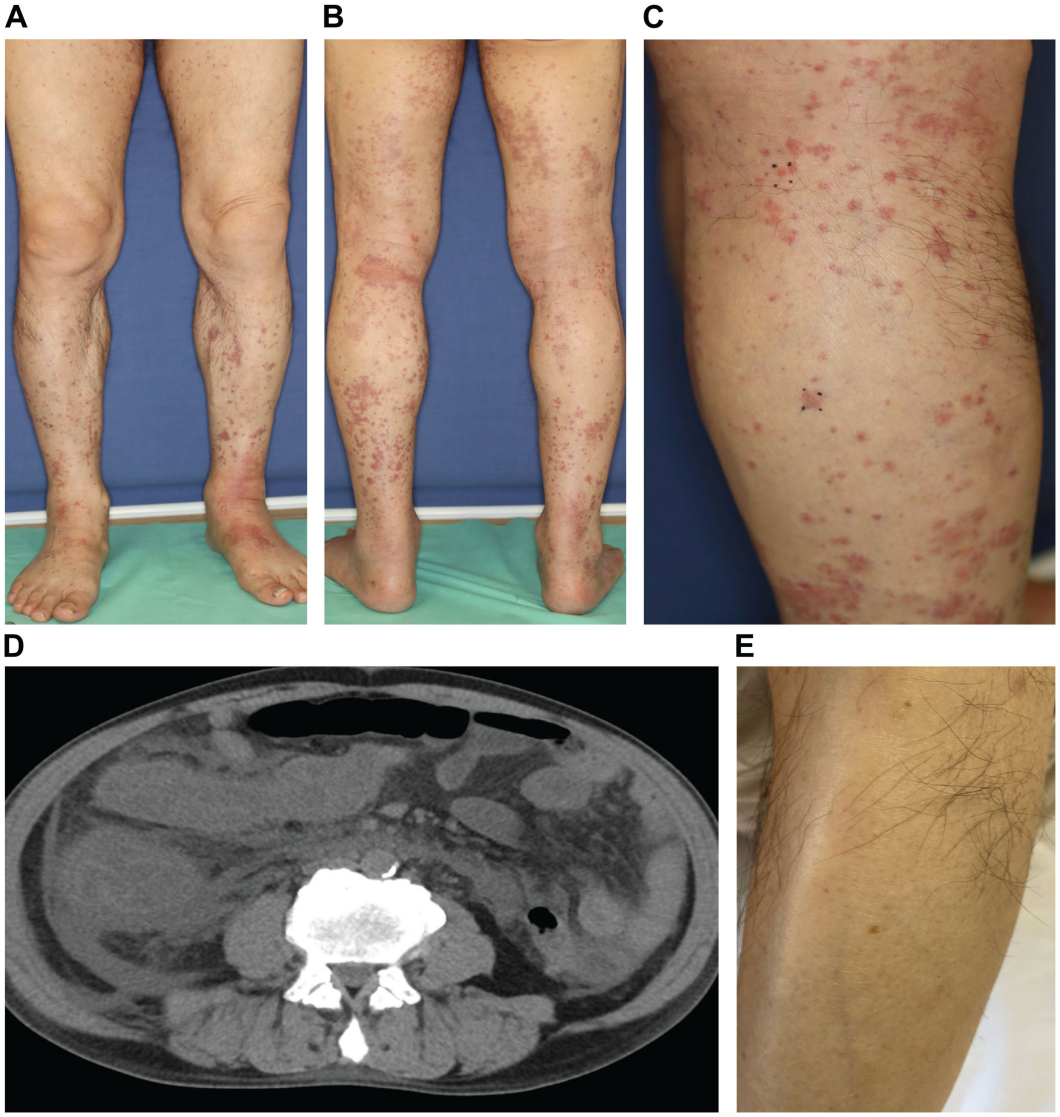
(A–C) The patient developed multiple purpuras on his extremities. (D) CT revealed intestinal edema and ascites. (E) The purpura improved immediately after glucocorticoid treatment.
